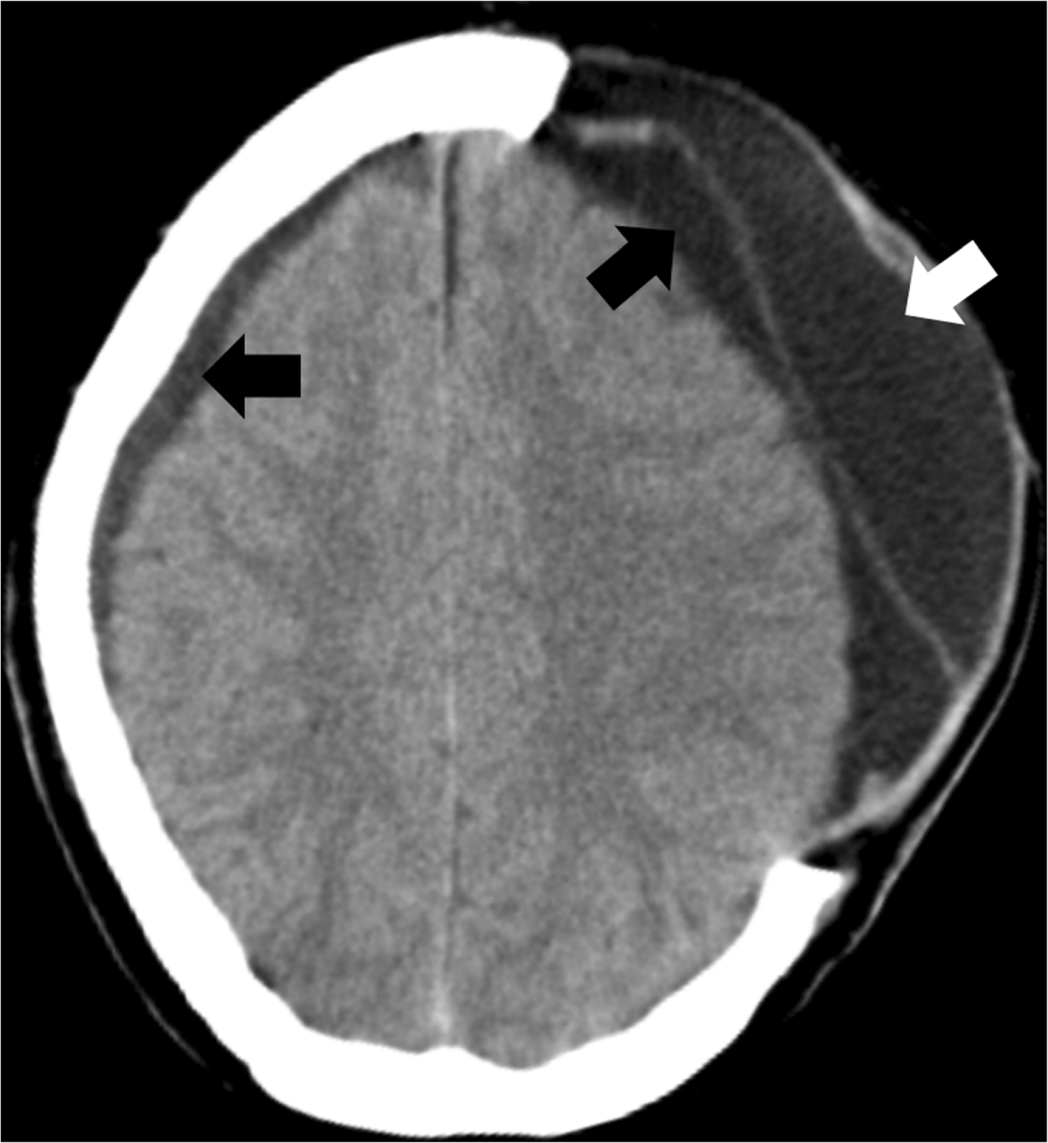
Hygroma occurring after DC. Axial CT scan showing ipsilateral and contralateral subdural hygroma (black arrows), which occurred after left-sided hemicraniectomy. Additionally, a large epidural fluid collection (white arrow) is observed
